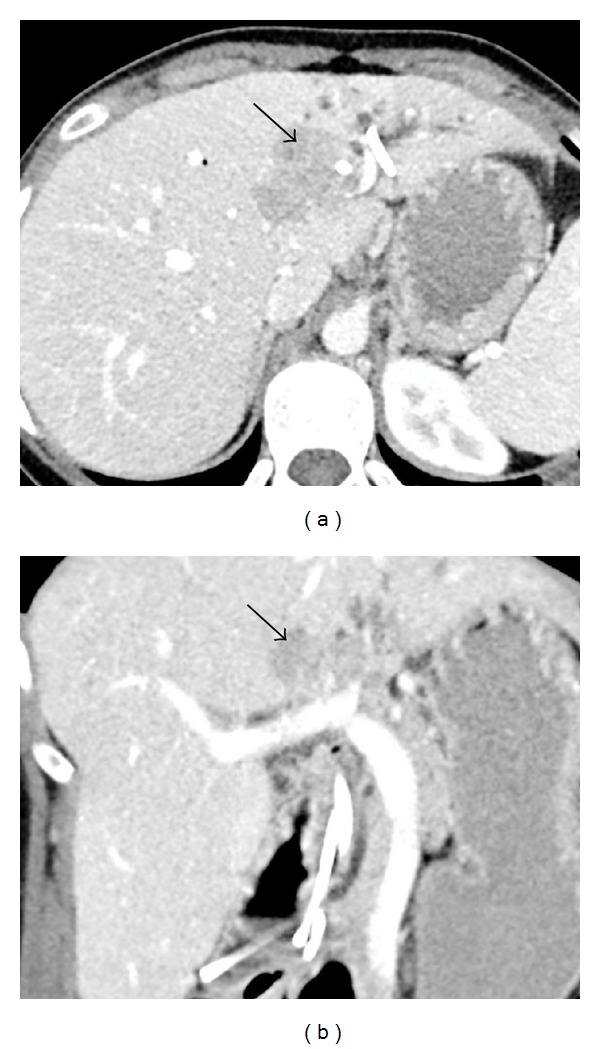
CT of the liver lesion (black arrow) in axial (a) and coronal (b) sections demonstrating an ill-defined heterogeneous soft tissue density in segment 4 as well as extensive intrahepatic biliary duct dilatation in segments 2 and 3 with pneumobilia.
